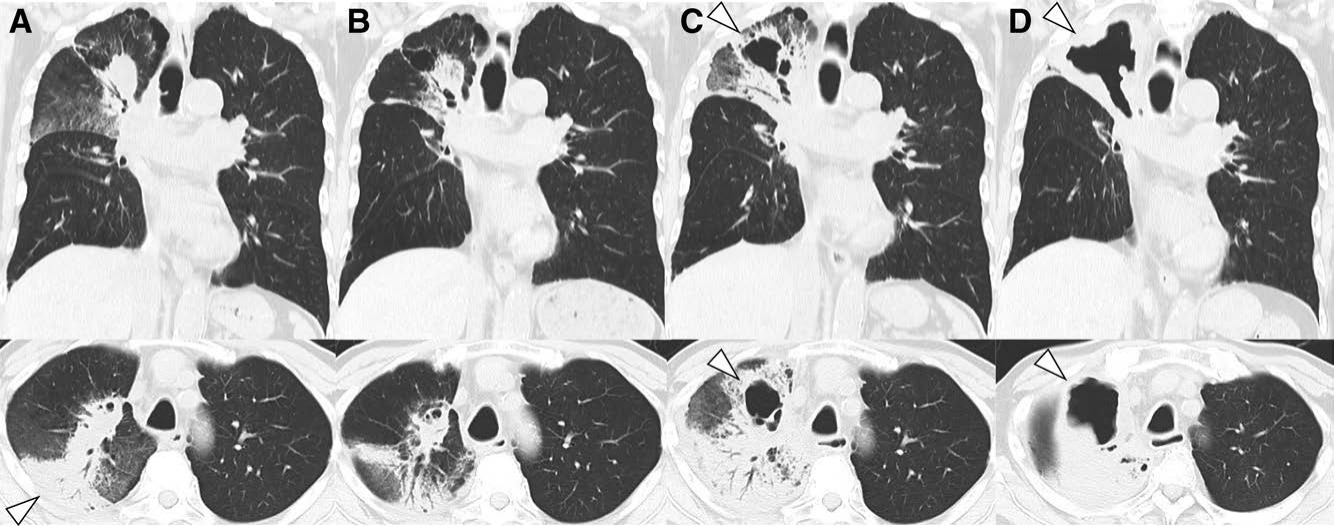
(A) Chest CT image after maintenance therapy with ipilimumab showing consolidation in the right upper lobe (arrowhead), raising suspicion for radiation/immune-related pneumonitis. (B) Improvement of consolidation after administering prednisolone (PSL) at 60 mg/d. (C) CT findings upon PSL reduction to 25 mg/d showing worsening infiltration with a cavitary lesion in the right upper lobe (arrowhead). These findings suggested relapse of pneumonia, either infectious or radiation/immune-related pneumonitis, prompting initiation of TAZ/PIPC and re-escalation of PSL. (D) Increased PSL to 60 mg/d and using antibiotic resulted in consolidation improvement; however, the cavitary lesion in the right upper lobe continued to enlarge (arrowhead).
